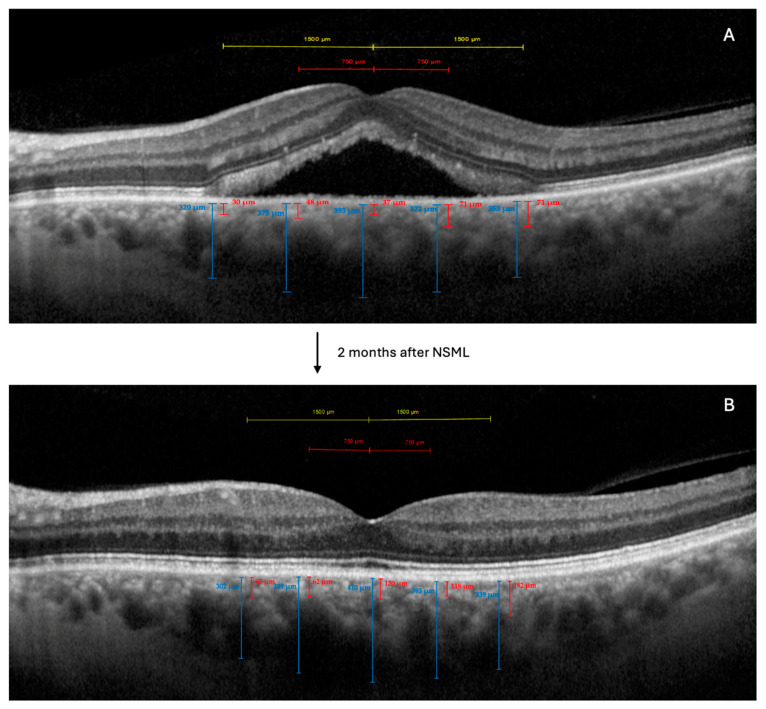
Enhanced-depth imaging optical coherence tomography (EDI-OCT) images of a patient with chronic central serous chorioretinopathy (cCSC), before and after navigated subthreshold micropulse laser treatment (NSML). (A): Baseline EDI-OCT image showing subretinal fluid (SRF) and choroidal thickness measurements at five locations: subfoveal, and at 750 μm and 1500 μm nasal and temporal to the fovea. (B): EDI-OCT image 2 months after SMLT, demonstrating resolution of SRF and changes in choroidal thickness. Note the increase in Sattler’s layer and choriocapillaris complex thickness (SLCCT) and total choroidal thickness (CT) compared to baseline. Vertical lines indicate choroidal thickness measurements. The upper portion of each line represents the outer border of the retinal pigment epithelium, while the lower end marks the choroidal–scleral interface.
